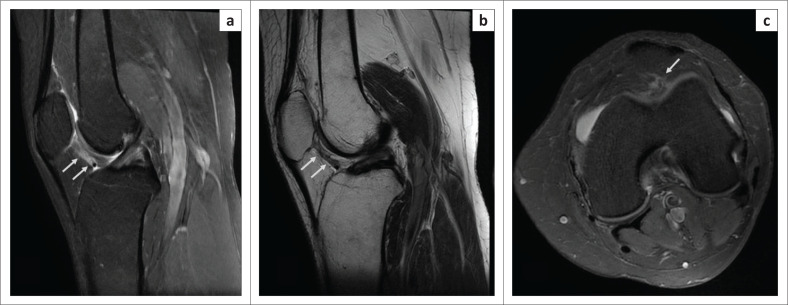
Magnetic resonance imaging (MRI) of infrapatellar plica sprain in a 50-year-old female with a history of twisting the knee: oblique sagittal PDFS image (a), T2WI (b) and axial PDFS image (c) indicate hyperintense signal along the entire course of infrapatellar plica (arrow), from the transverse inter-meniscal ligament to the inferior pole of patella, with its margins appearing ragged. Note the signal is less hyperintense than that of the synovial fluid. No other abnormality was noted on the patient’s knee MRI.
